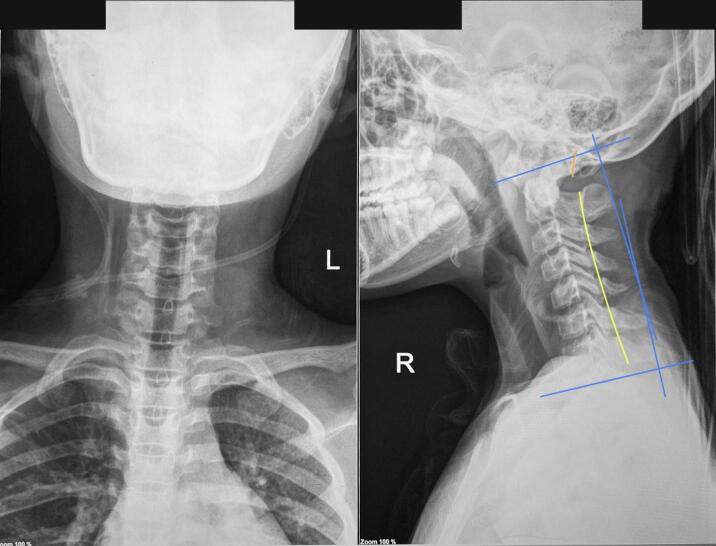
Cervical X-Ray in AP and Lateral view on the patient showing normal cervical anatomy without fracture nor listhesis.
